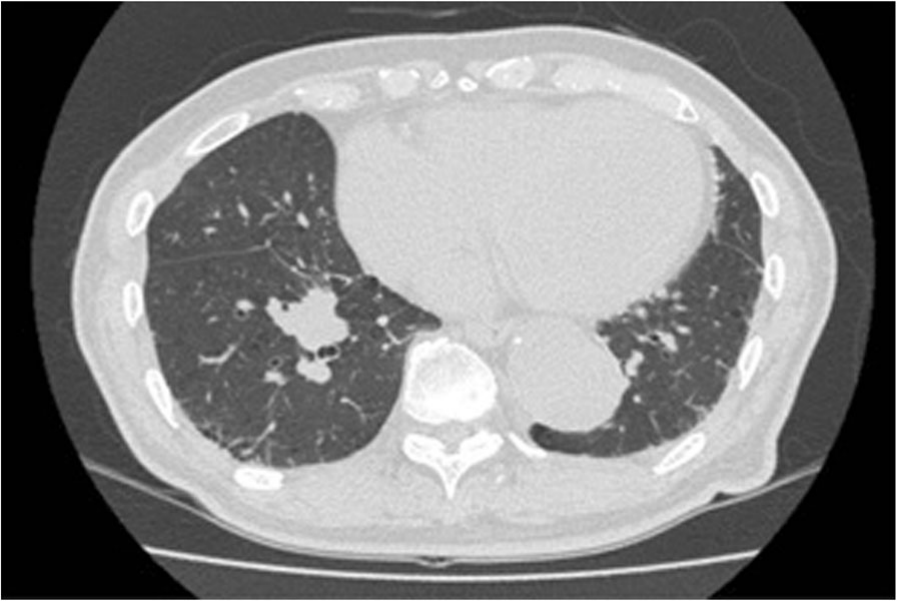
Axial image of a chest computed tomography (CT) scan
